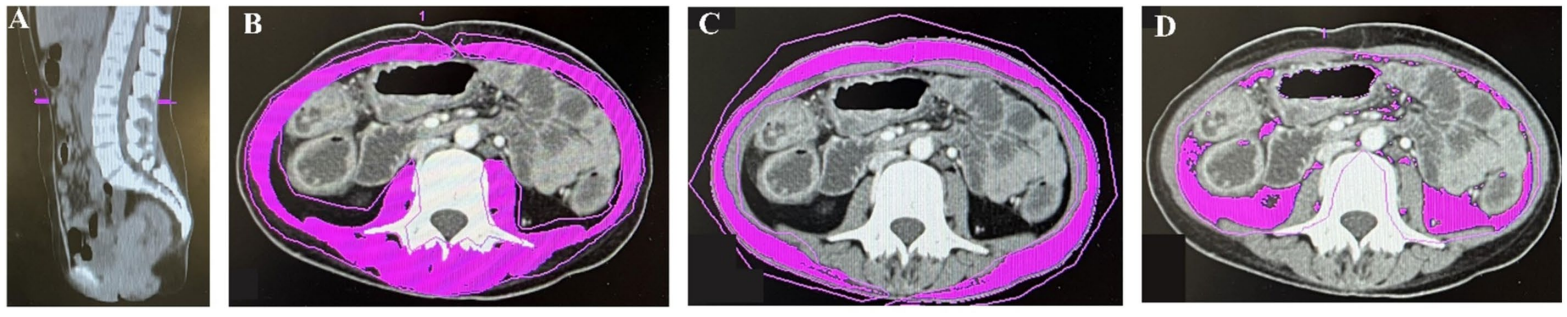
The midsagittal image in computed tomography (CT) was used to find the third lumbar vertebrae (A). Skeletal muscle area (SMA) (B), subcutaneous fat area (SFA) (C), and visceral fat area (VFA) (D) were measured on CT images.
